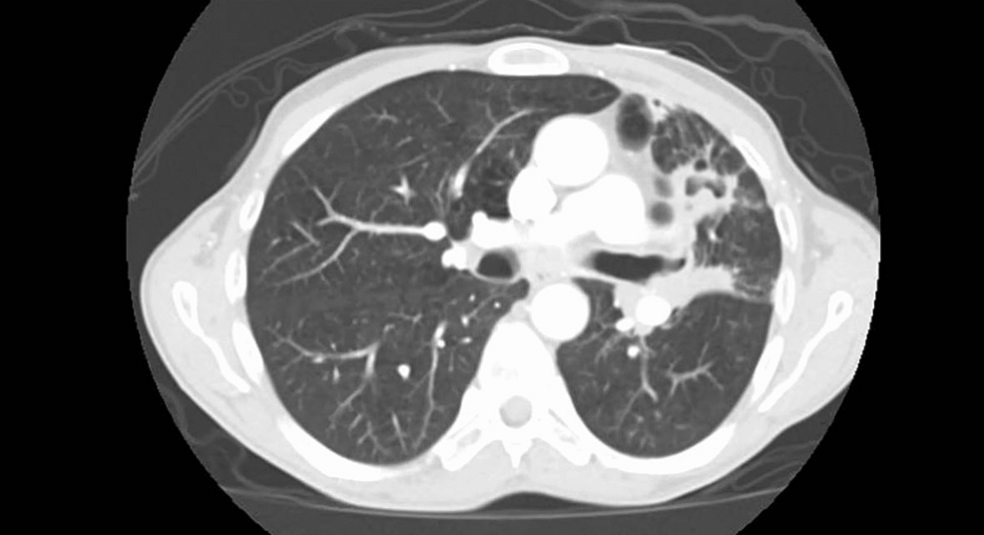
CT of the chest showing tree-in-bud opacities bilaterally
